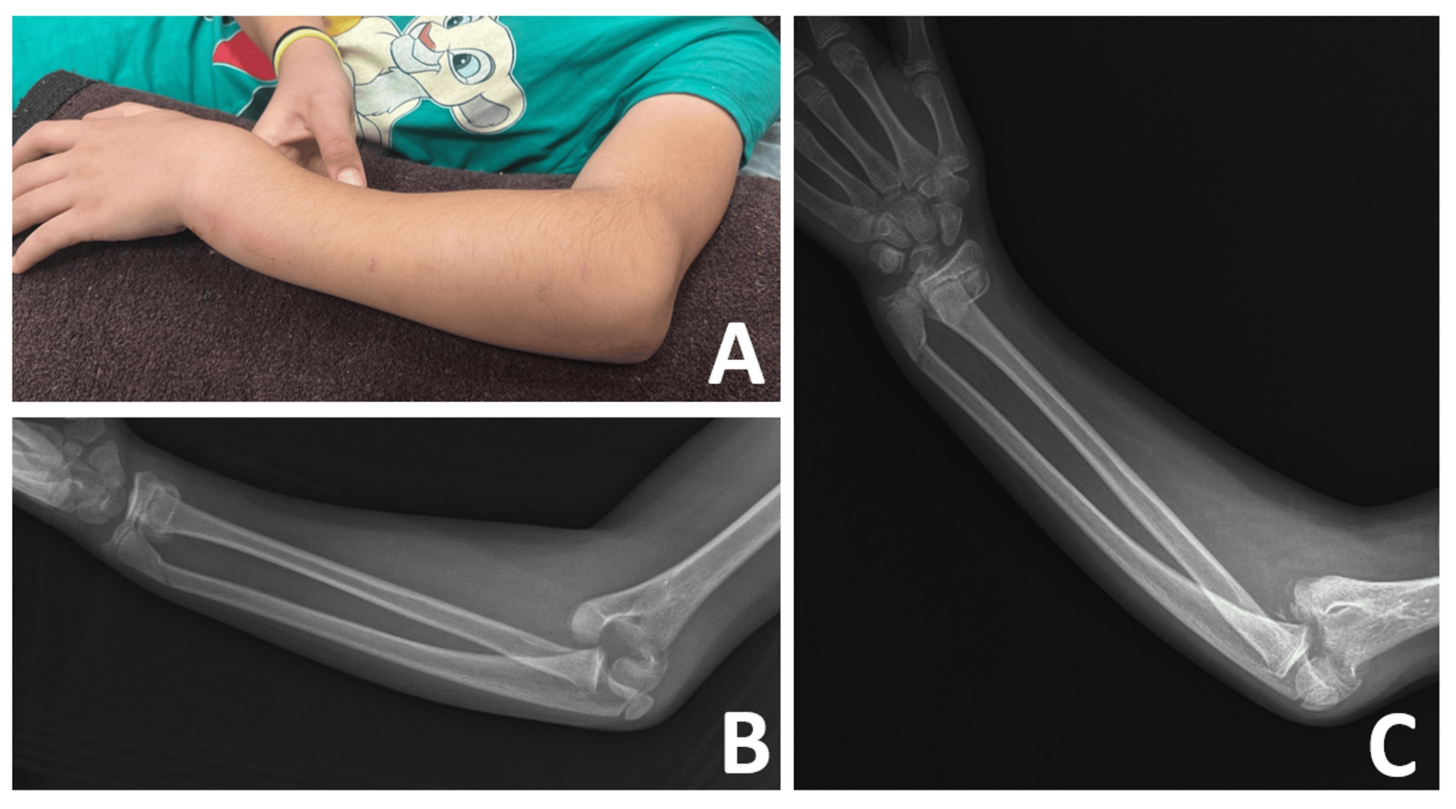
Initial photograph and radiographs of the left upper extremity in the emergency department (A) The photograph taken by the emergency physician shows deformities in the left elbow and wrist. (B and C) Initial radiographs demonstrate distal both-bone forearm and lateral humeral condyle fractures with posterolateral elbow dislocation.
